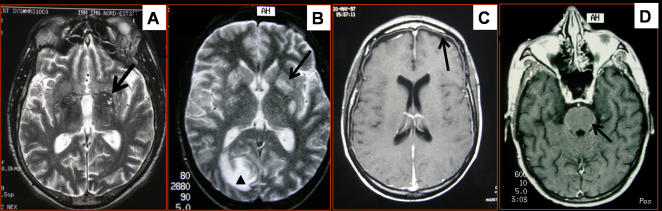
Examples of abnormal radiological findings. A. Magnetic Resonance axial T2-weighted image, displaying bilateral dilated Virchow-Robin spaces (arrow) in the basal ganglia. B. Magnetic Resonance axial T2-weighted image displaying a hyperintense right occipital mass (arrow head) and bilateral dilated Virchow-Robin spaces (arrow). C. Magnetic Resonance axial T1-weighted image with contrast infusion displaying frontal subdural collection (arrow). D. Magnetic Resonance axial T1-weighted image with contrast infusion displaying a basal meningeal enhancement (arrow).
